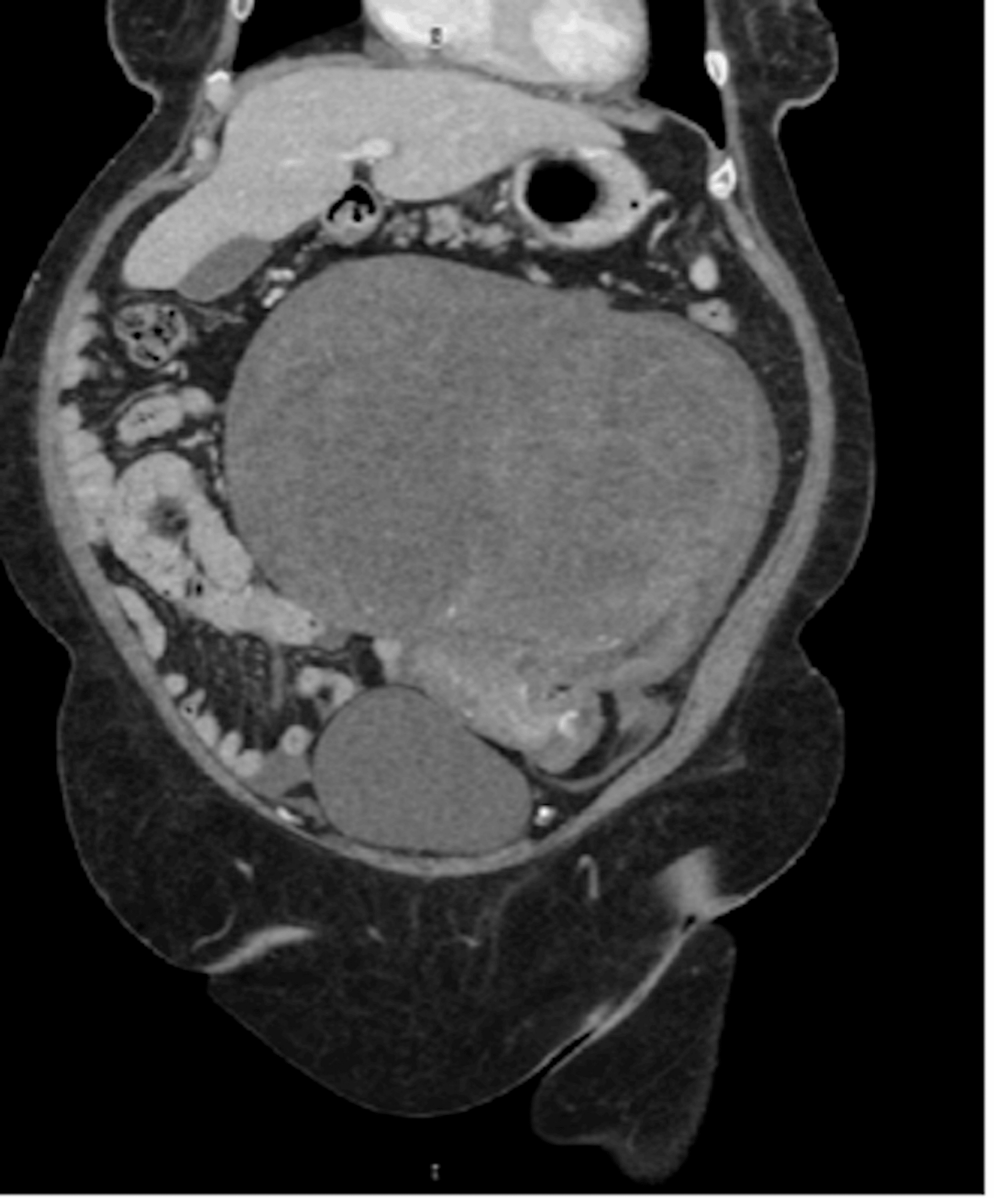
Large pelvic mass on CT imaging
